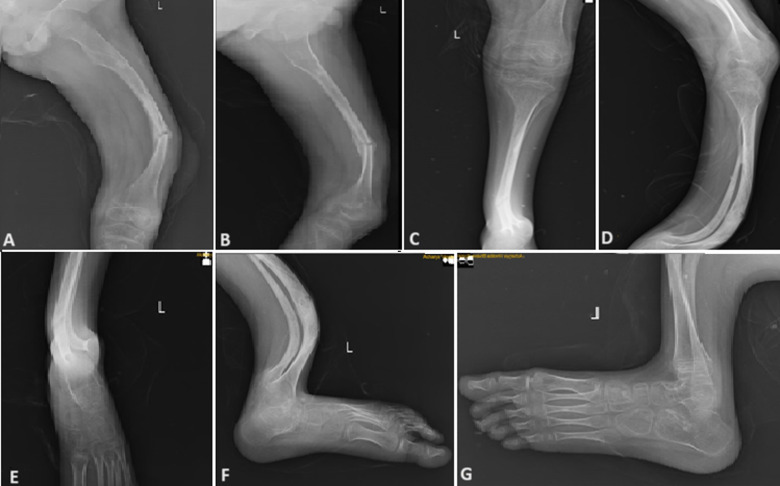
(A, B) X-ray suggestive of anterior bowing deformity of femur with old malunited femur fracture left side; (C, D) X-ray suggestive of anterior bowing deformity of both tibia and fibula left side; (E, F, G) X-ray suggestive of abnormal growths of tarsal bones present
